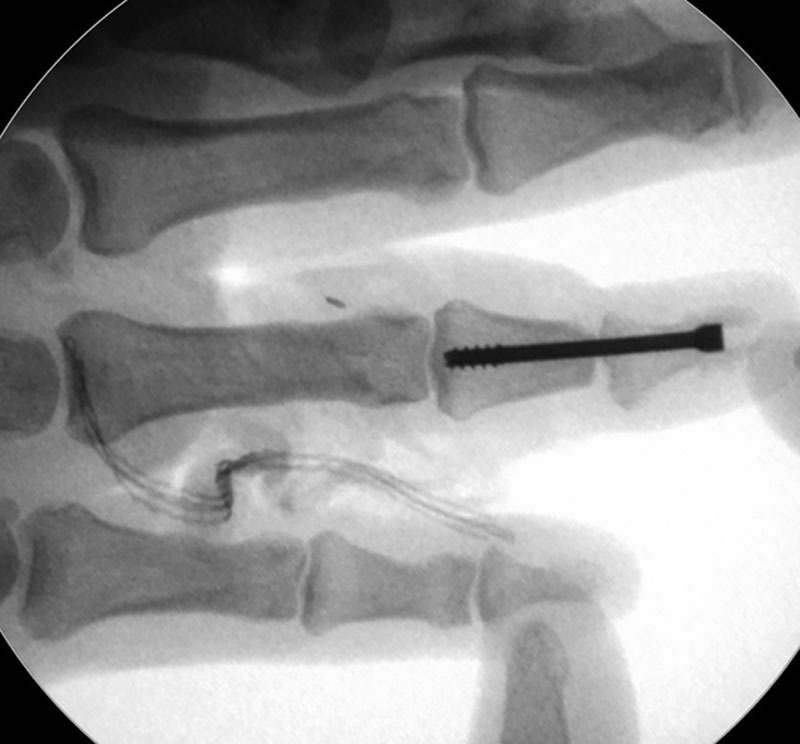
Intraoperative radiograph showing 2-mm axial screw fixation, achieving satisfactory anatomic distal phalanx alignment.
